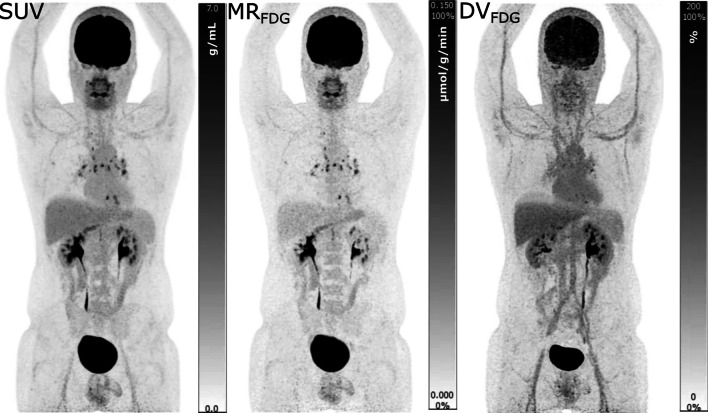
Example of SUV and Patlak images analysed in our study. In this case, a 34-year-old man with mediastinal sarcoidosis. Left column: static SUV images; Middle column: MR_FDG_ images; right column: DV_FDG_ images. The actual FDG uptake in the inflammatory sarcoid lymph nodes is evident on the MR_FDG_ image, whereas the free FDG in the circulation is clearly visible on the DV_FDG_ image
